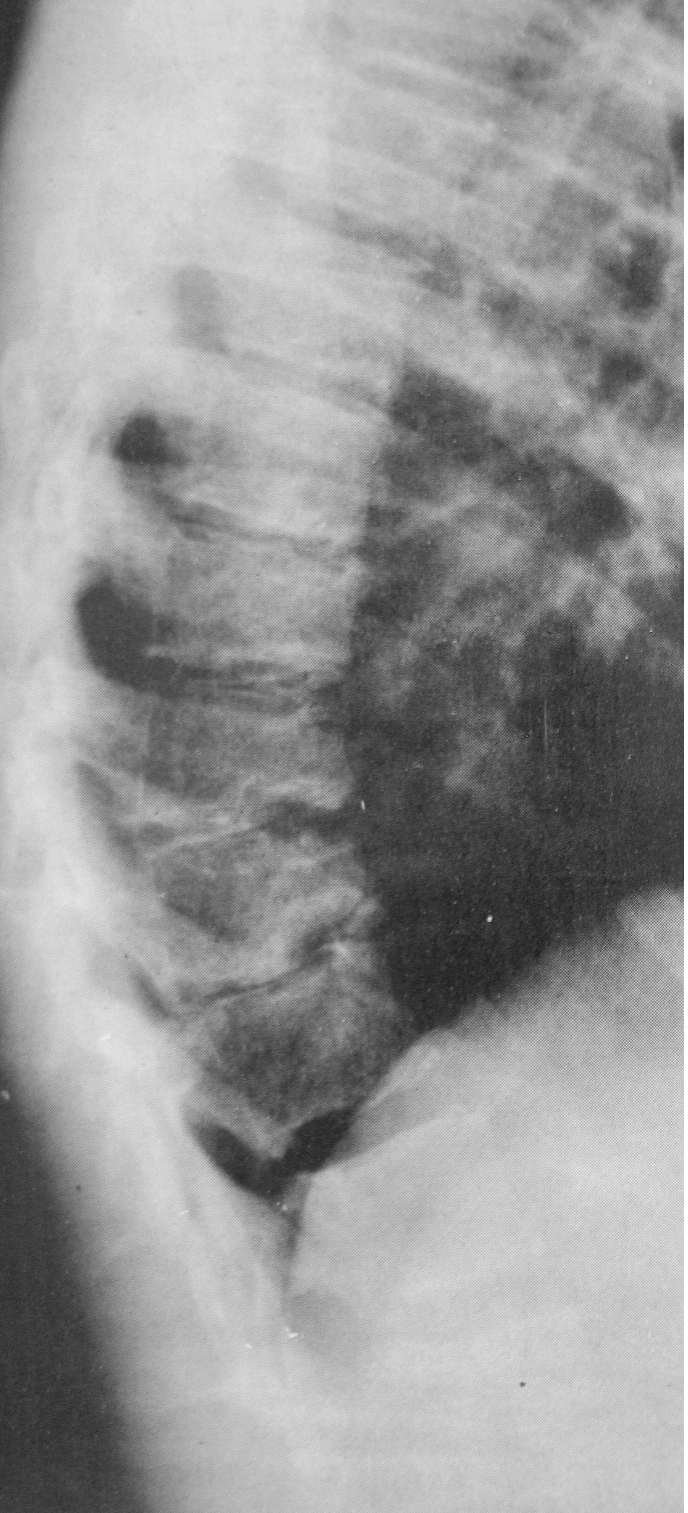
Scheuermann signs on a lateral X-ray in the thoracolumbar region. The Schmorl's nodes and endplate irregularity may be so severe that lumbar Scheuermann's disease can be confused with infection, tumor, or other conditions [8].
